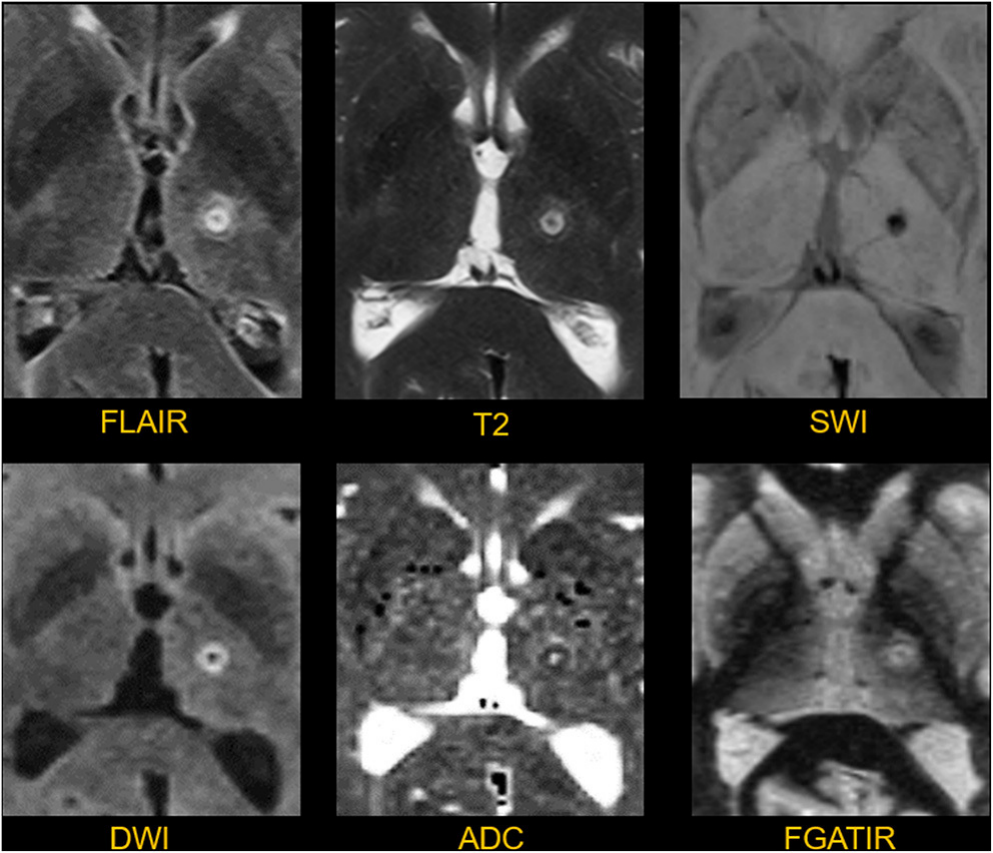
Appearance of a thalamic lesion on postoperative day 1. The lesion in the left thalamus is manifest as a T2/FLAIR spherical hyperintense focus with a diameter of about 6 mm. There is hemosiderin clearly shown in the susceptibility weighted image (SWI). There is a rim of restricted diffusion with a core of facilitated diffusion shown on the DWI and ADC images. The FGATIR sequence shows the relationship between the lesion, thalamus, and adjacent internal capsule.
